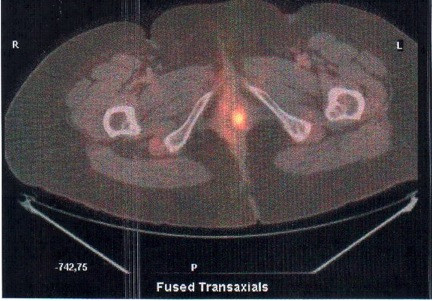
Isolated lesion to left vaginal wall at Total Body CT-PET scan.
